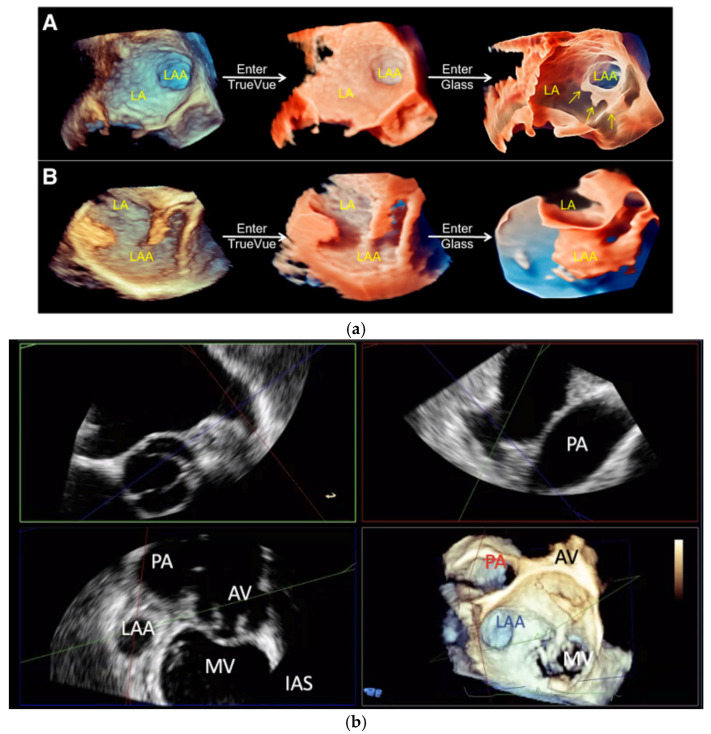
(a) Conversion of conventional real-time 3D transoesophageal echocardiography to TrueVue Glass. (A) From left to right, conventional 3D, TrueVue, and TrueVue Glass imaging modes of the LAA at the entrance of the left atrium are shown. The TrueVue Glass (right) shows the three lobular structures at the blind end of the LAA (arrows). (B) From left to right, conventional 3D, TrueVue, and TrueVue Glass imaging of the LAA in lateral view are shown. 3D: three-dimensional; LA: Left atrium; LAA: Left atrial appendage. Adapted with permission from Sun et al. [27]. (b) Three-dimensional (3D) multiplanar reformat images depicting the anatomical relationship of the LAA with the surrounding structures as seen with 3D TOE. AV: Aortic valve; MV: Mitral valve; PA: Pulmonary artery; LAA: Left atrial appendage; IAS: Inter-atrial septum; TOE: Transoesophageal echocardiogram.
